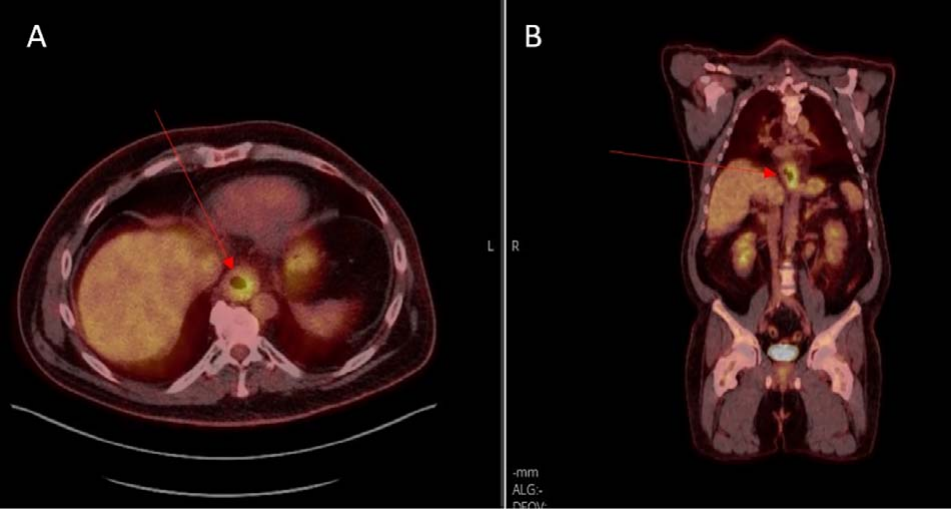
(A) Axial fused positron emission tomography-computed tomography (PET-CT) shows the hypermetabolic mass (arrow) at the distal esophagus (standardized uptake value maximum 18). (B) Coronal fused PET-CT shows the hypermetabolic mass (arrow) at the distal esophagus and extending to the gastroesophageal junction. The patient had regional lymphadenopathy but no evidence of distant metastases.
